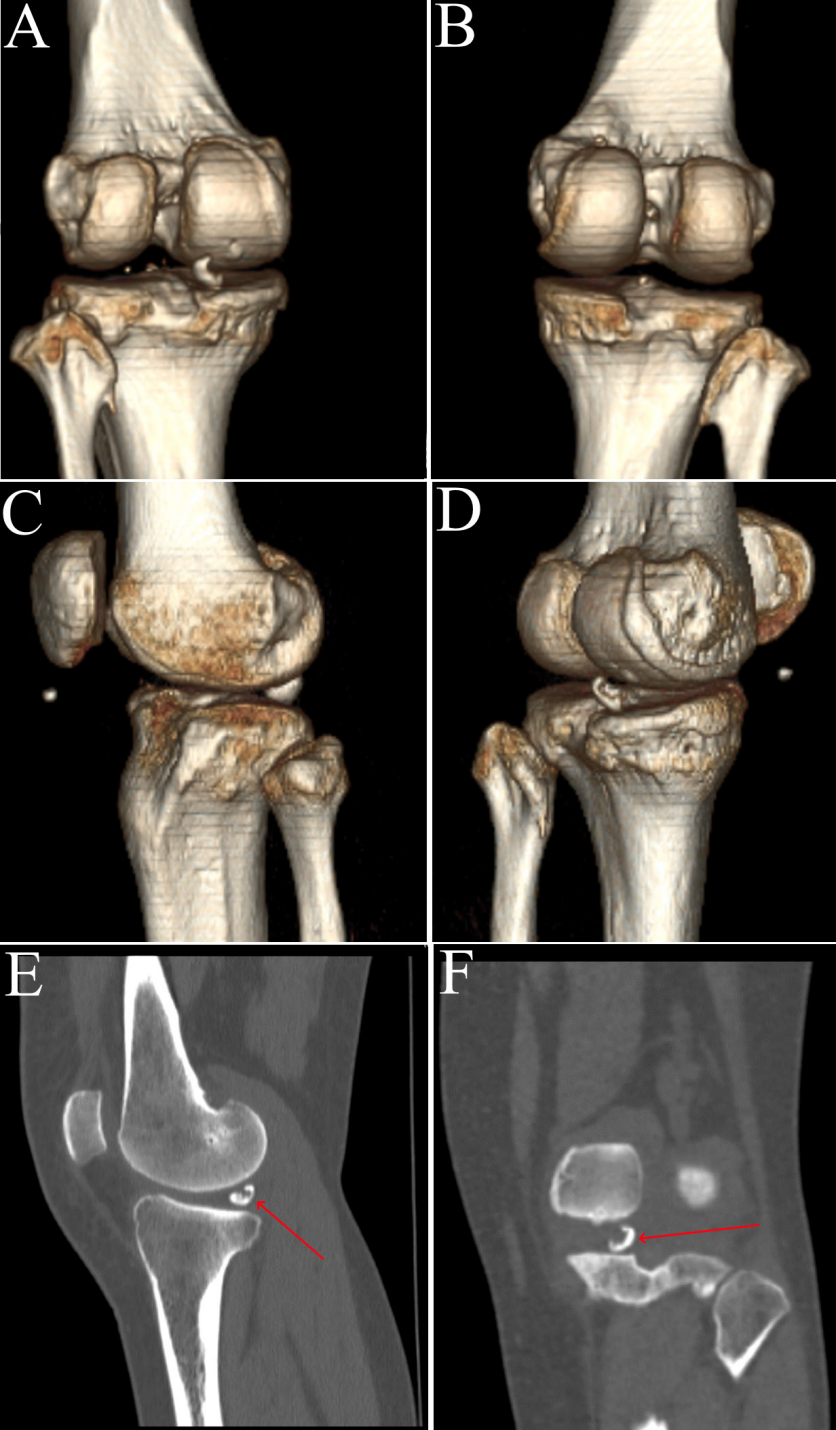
Sagittal CT scan (A-D) 3D model generated from CT scan showing meniscal ossicle (E-F) meniscal ossicle (straight arrow). 3D, three-dimensional.
